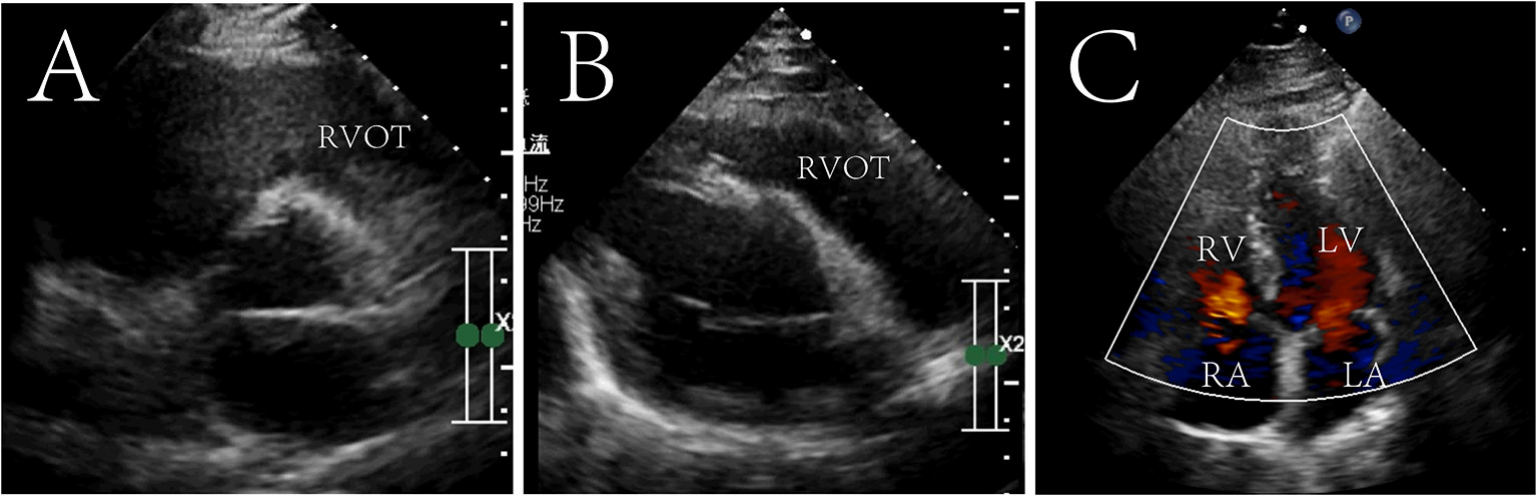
Seven-year postoperative echocardiography in transthoracic aortic short-axis views (A), right ventricular outflow tract view (B) and four chamber cross section view (C). The tumor did not recur and the right ventricular outflow tract was not obstructed. Right ventricular outflow tract, RVOT; RV, right ventricle; LV, left ventricle; RA, right atrium; LA, left atrium.
